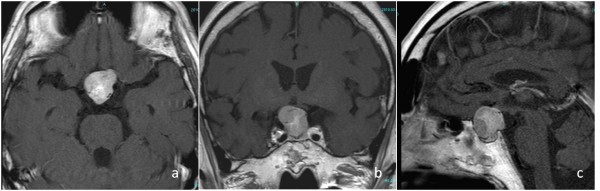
Magnetic resonance imaging at 7 years after initial surgery. A–C) Axial, coronal and sagittal contrast-enhanced magnetic resonance image showing enlargement of the tumor.
